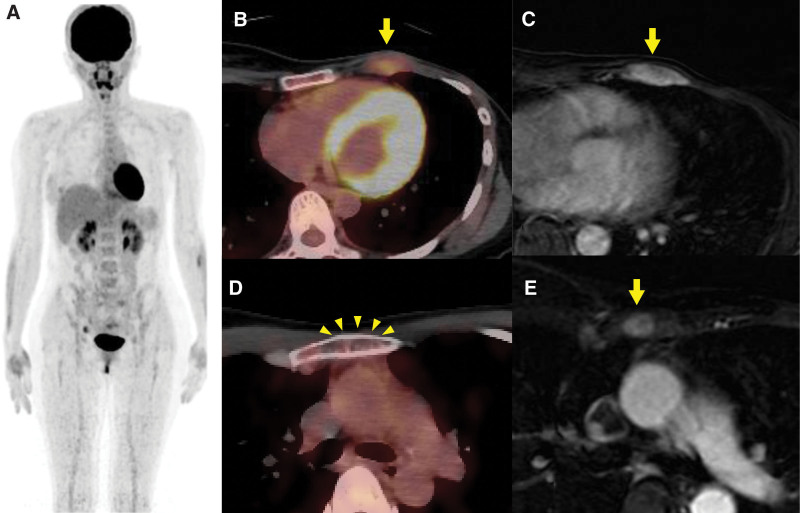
A 37-year-old woman was diagnosed with primary angiosarcoma of the breast and underwent mastectomy. During the postoperative follow-up, although the uptake was covered by the heart in the maximum intensity projection image (A), axial fluorine-18 fluorodeoxyglucose positron emission tomography/computed tomography (^18^F-FDG PET/CT) revealed abnormal uptake (SUVmax = 3.1) in the left third intercostal muscle (yellow arrow) (B). Gd-enhanced magnetic resonance imaging (MRI) also showed soft tissue mass in the left intercostal muscle (yellow arrow) in the axial image, suggesting local recurrence (C). Furthermore, axial Gd-enhanced MRI showed a 13-mm (diameter) contrast effect in the sternum (yellow arrow) (E), which suggested bone metastasis. Contrary to these findings, axial ^18^F-FDG PET/CT showed no uptake or osteolytic destruction in the sternum (D). SUV = standardized uptake value.
